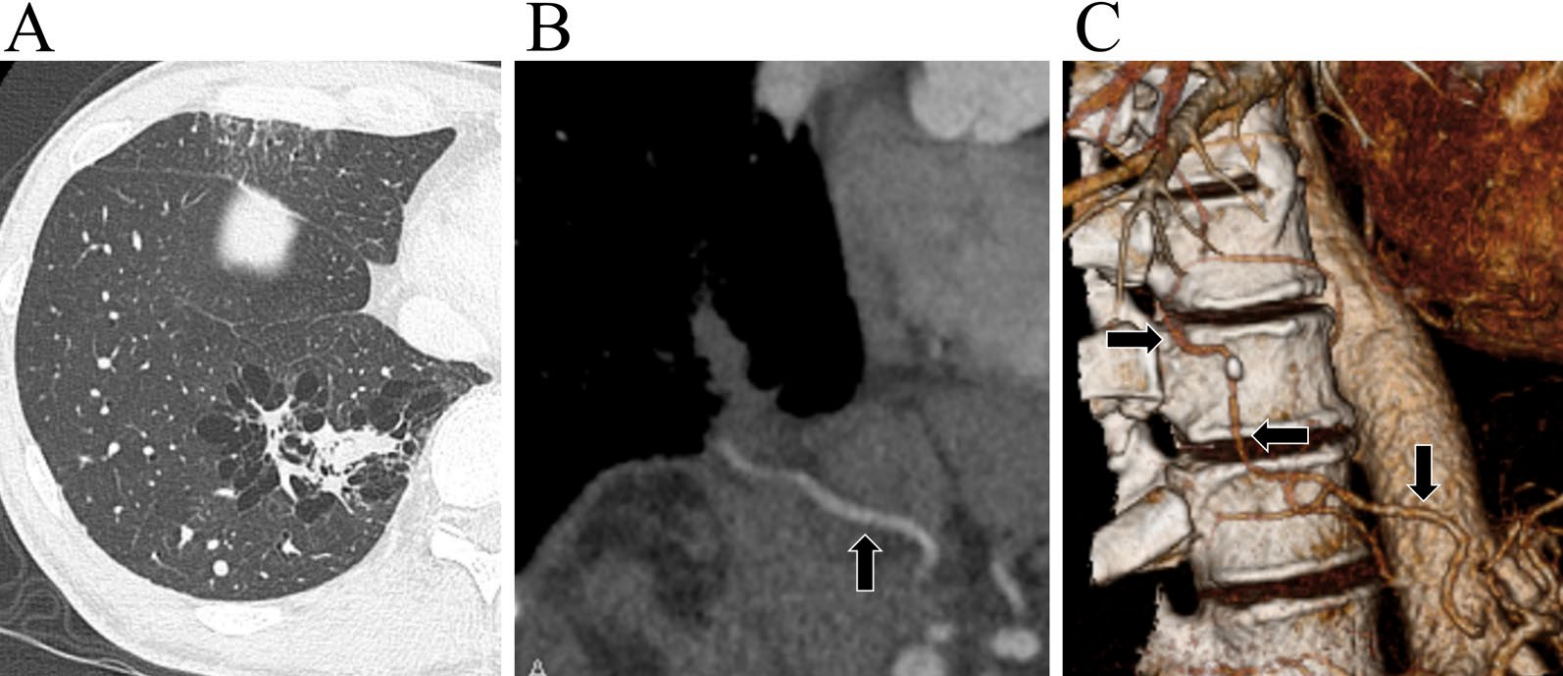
Preoperative image of enhanced computed tomography and three-dimensional computed tomography. A Enhanced computed tomography (CT) scan showing intralobar sequestration. B Enhanced CT scan reveals an abnormal artery branching from the abdominal aorta (arrow). C Three-dimensional CT scan reveals an abnormal artery branching from the abdominal aorta (arrow)
